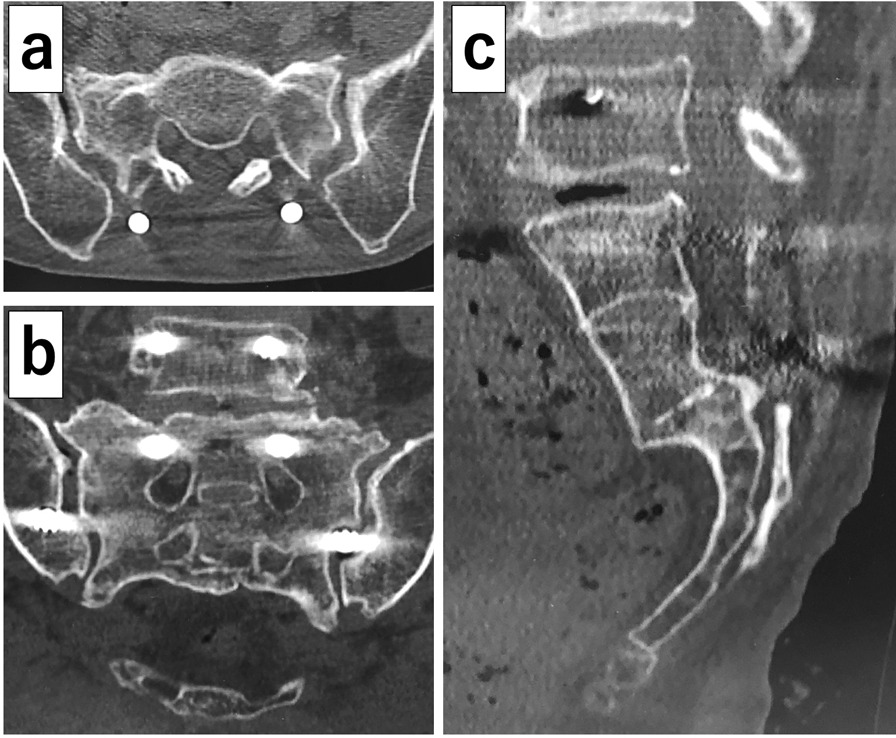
Postoperative computed tomography 3 months after surgery. a Axial image; b coronal reconstruction image; c sagittal reconstruction image. Bone fusion is observed in all images
